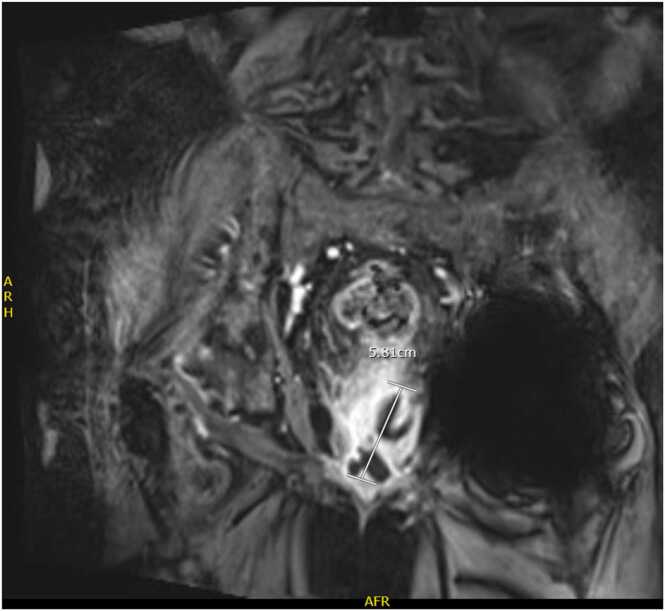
MRI of the abdomen and pelvis shows a demonstration of a 3.9 × 2.8 × 5.8 cm collection with irregular, thick enhancing walls arisen from the left peripheral zone mid base of the prostate gland.
